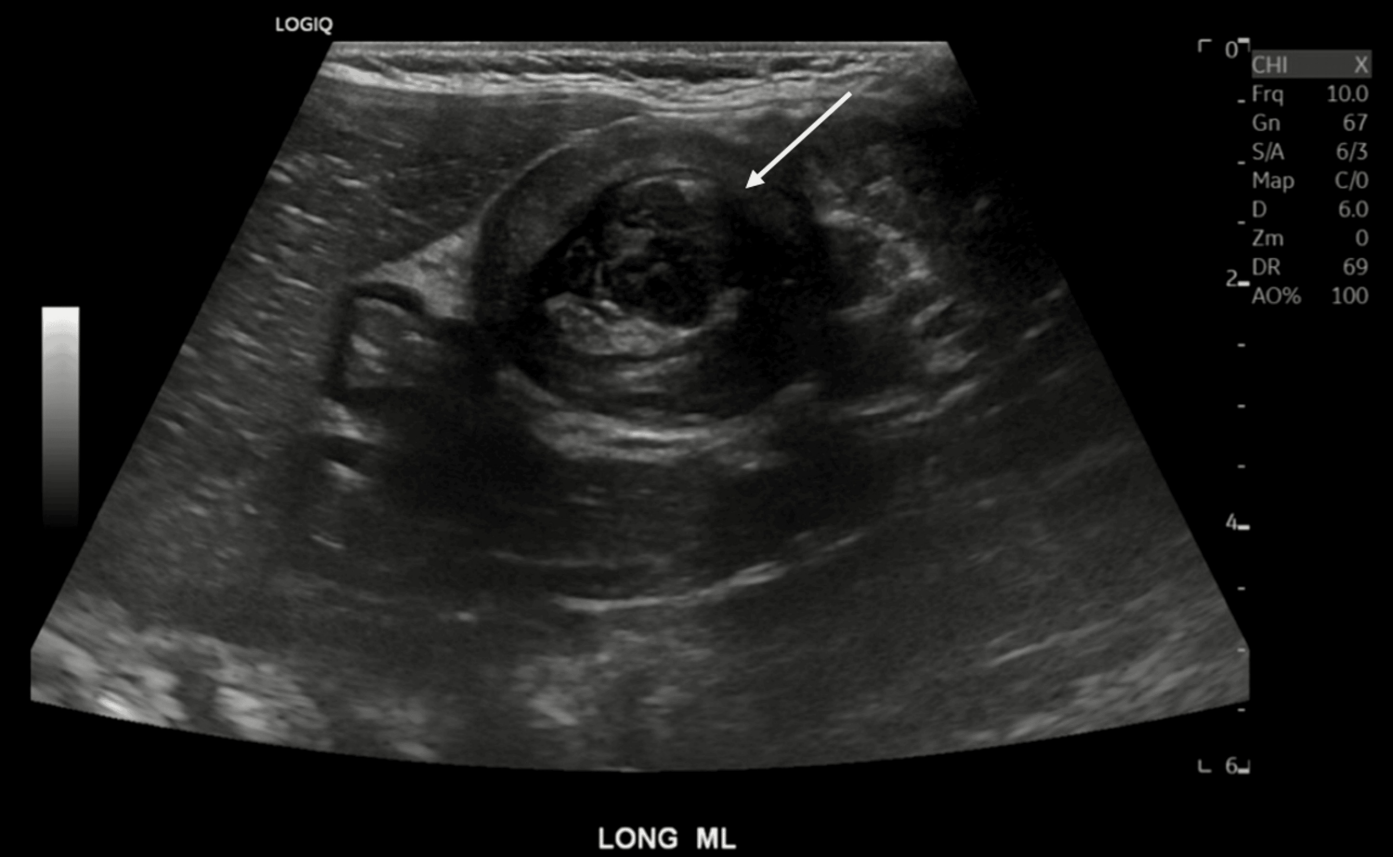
Ileocolic intussusception on abdominal ultrasound (midline long-axis view)
